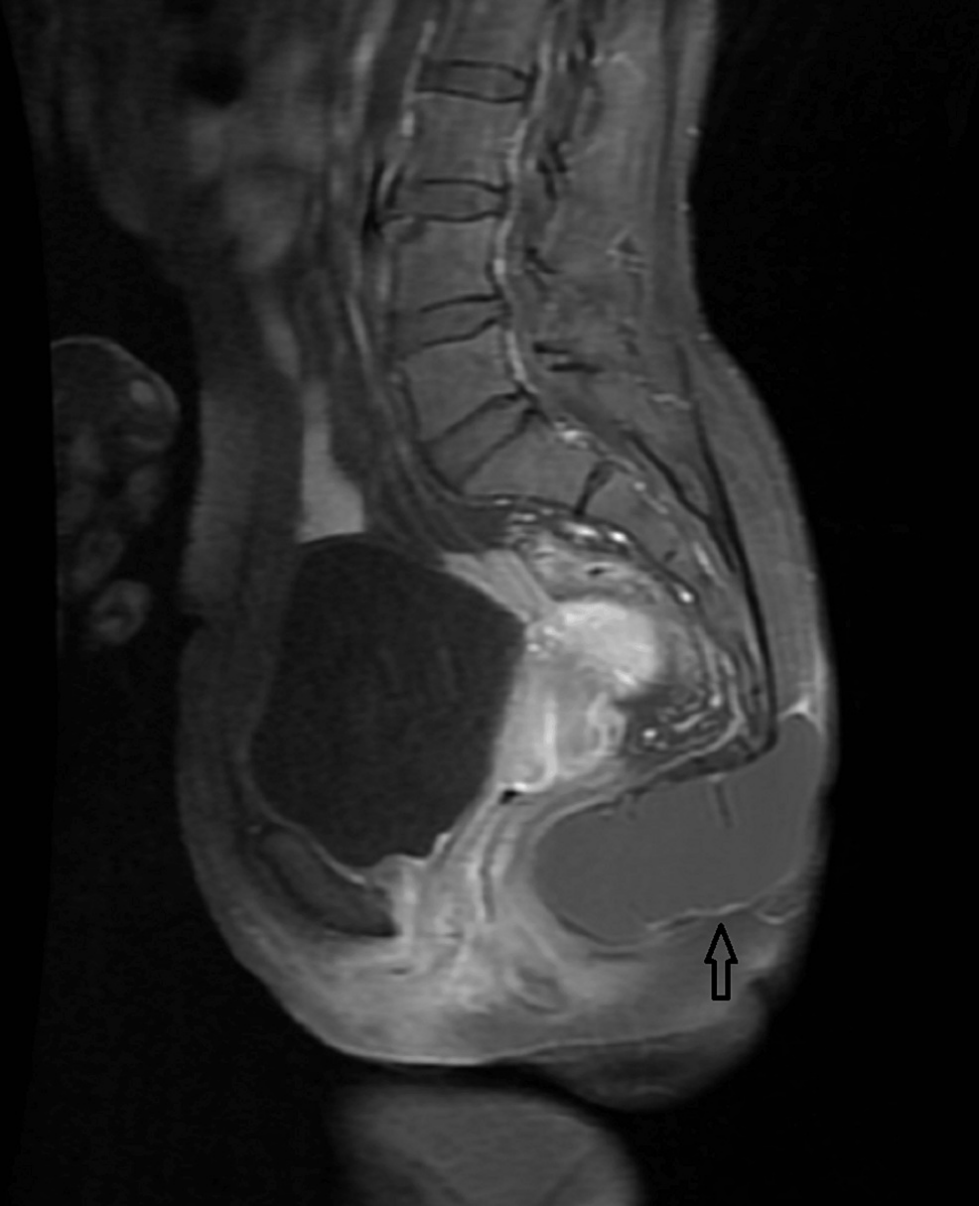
Contrast-enhanced T1WI sagittal MRI image showing subtle peripheral enhancement (black arrow). T1WI: T1 weighted image https://assets.cureus.com/uploads/figure/file/450604/lightbox_d7c86760313b11ed8c9887c19bb9f4af-E5.png
